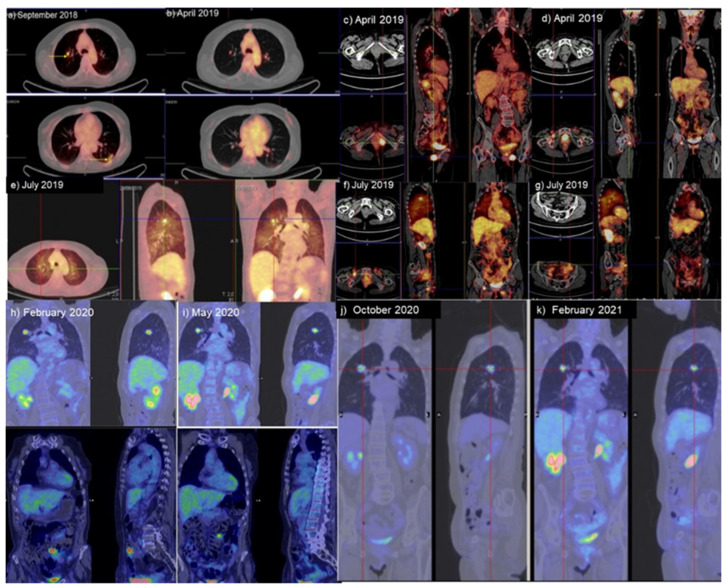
PET/CT during disease course: (a) In September 2018, PET/CT showed the appearance of hypermetabolic bilateral lung nodules; (b) the PET/CT scans of April 2019 after 6 months of Nivolumab showed a significant reduction in and disappearance of hypermetabolic activity of the lung lesions; (c,d) PET/CT scan on April 2019 showed stable hypermetabolic activity in the left perineum and vagina and hypermetabolic activity in the right inguinal region; (e–g) in July 2019, imaging showed progression of disease in the lung (e) in comparison to April 2019, as well as the appearance of hypermetabolic areas in the left anterior abdominal wall (f) and in the right inguinal region (g); (h,i) PET/CT imaging on February (h) and May 2020 (i) after 6 and 9 months of treatment with mTOR inhibitor, respectively; (j,k) PET/CT imaging in October 2021 and February 2021 before and after 3 months of treatment with Palbociclib.
